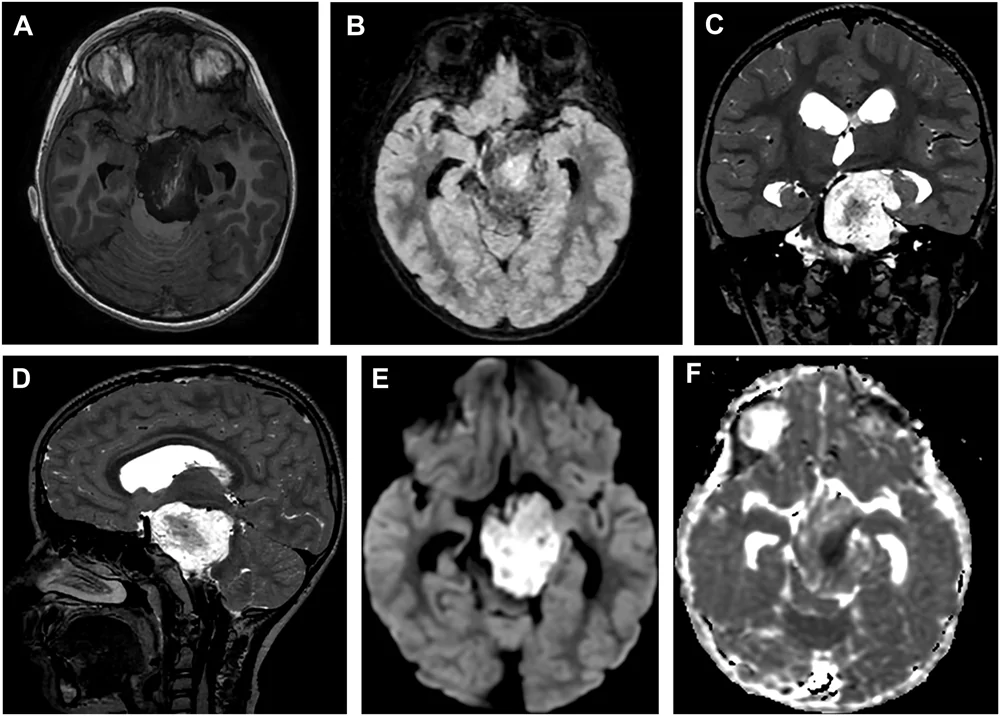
MRI demonstrates a well-defined extra-axial lesion in the petroclival region without contrast enhancement on axial T1-weighted imaging after gadolinium administration (A). The lesion exhibits incomplete suppression on axial fluid-attenuated inversion recovery (B) and displays a heterogeneous signal on coronal (C) and sagittal (D) T2-weighted imaging, characterized by central hypointense areas. Diffusion-weighted imaging (E) reveals marked restriction, with corresponding low signal on the apparent diffusion coefficient map (F). The overall heterogeneous appearance, with mixed T1 and T2 signal characteristics, is consistent with a dermoid cyst. This figure is original to this submission, so no credit or license is needed.
